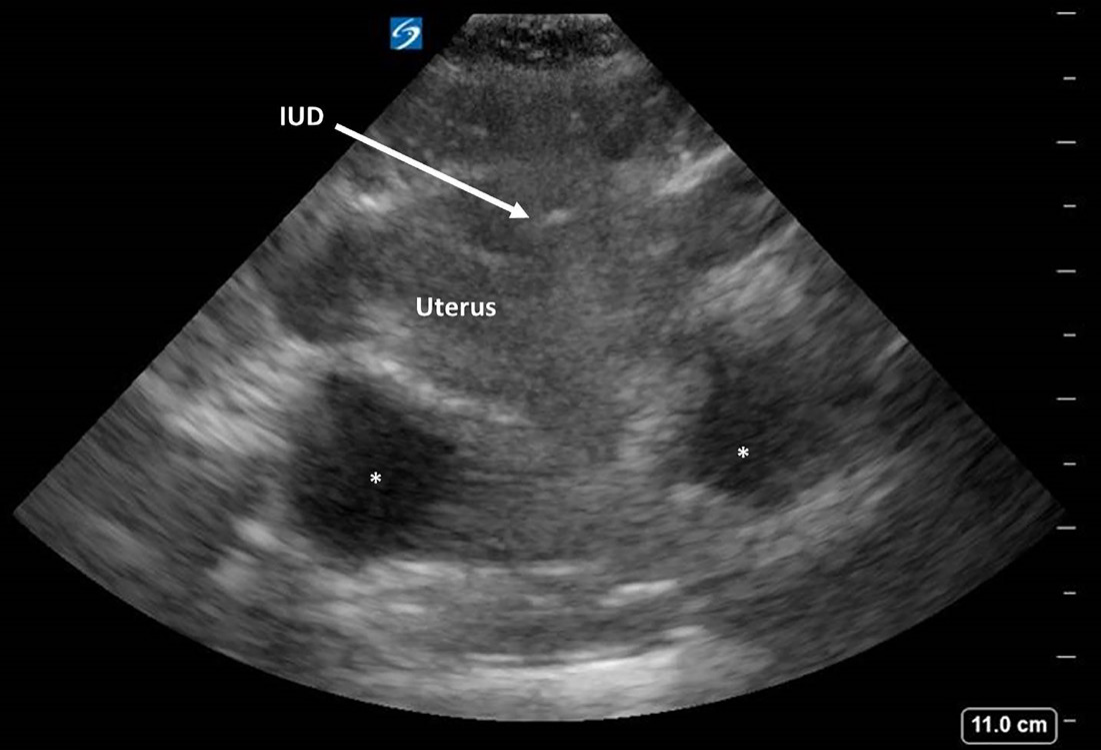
Transabdominal point-of-care ultrasound of the pelvis demonstrating an intrauterine device within the uterus (arrow), no evidence of intrauterine pregnancy, and free fluid posterior to the uterus (asterisks).
